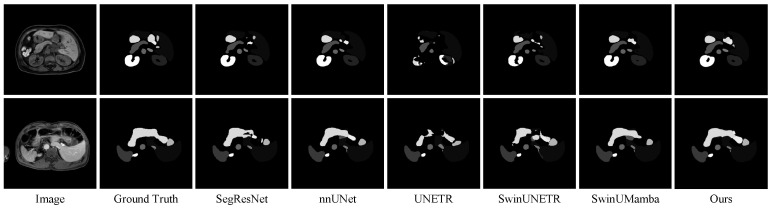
Visualizations on the Abdomen MRI dataset.
